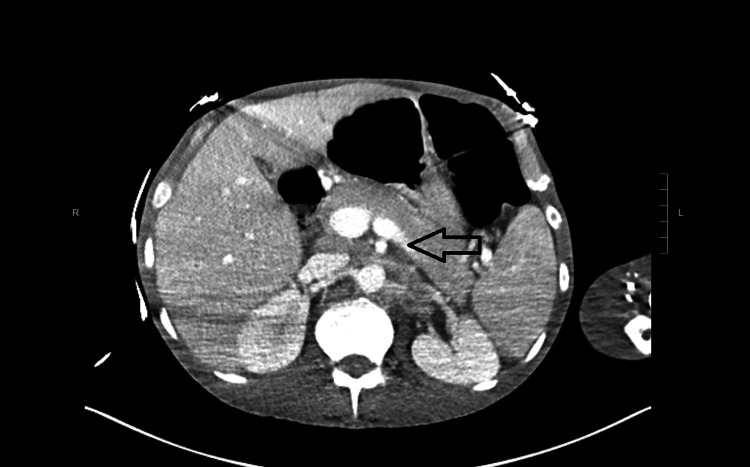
CT abdomen showing extensive retroperitoneal and pelvic adenopathy.
